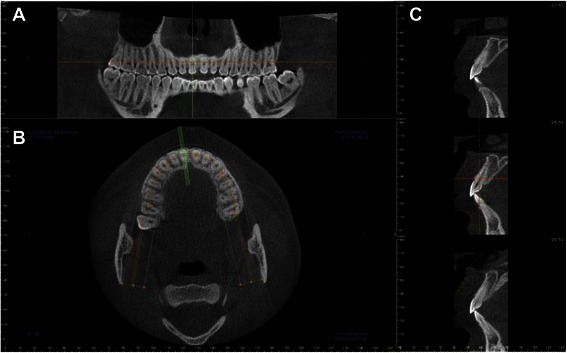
Reformatted CBCT views. a. Reformatted panoramic view demonstrates that the occlusal plane is parallel to the floor. b. Axial view at the maxillary arch level. The green lines are perpendicular to alveolar ridge. They indicate where the cross sectional views were taken. c. Series of cross sectional views. The view in the middle panel (corresponding to the middle green line in B) was used for alveolar volume and buccal undercut measurements
